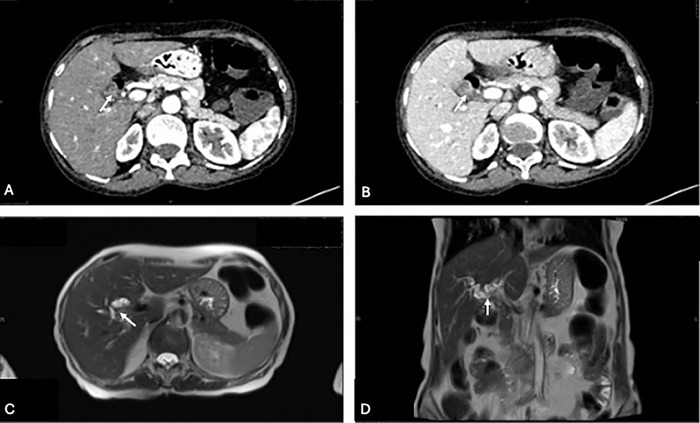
(A, B) Contrast-enhanced computed tomography of the abdomen shows a focal nodular enhancing lesion (arrows) involving the proximal common hepatic duct. (C) Axial T2-weighted magnetic resonance image shows an ill-defined isointense to hypointense lesion (arrow) at the proximal common hepatic duct. (D) Magnetic resonance cholangiopancreatography image shows focal narrowing of the proximal common hepatic duct (arrow) with upstream dilatation of the right hepatic duct, left hepatic duct, and intrahepatic biliary radicles, raising suspicion for a Bismuth-Corlette type I hilar cholangiocarcinoma.
